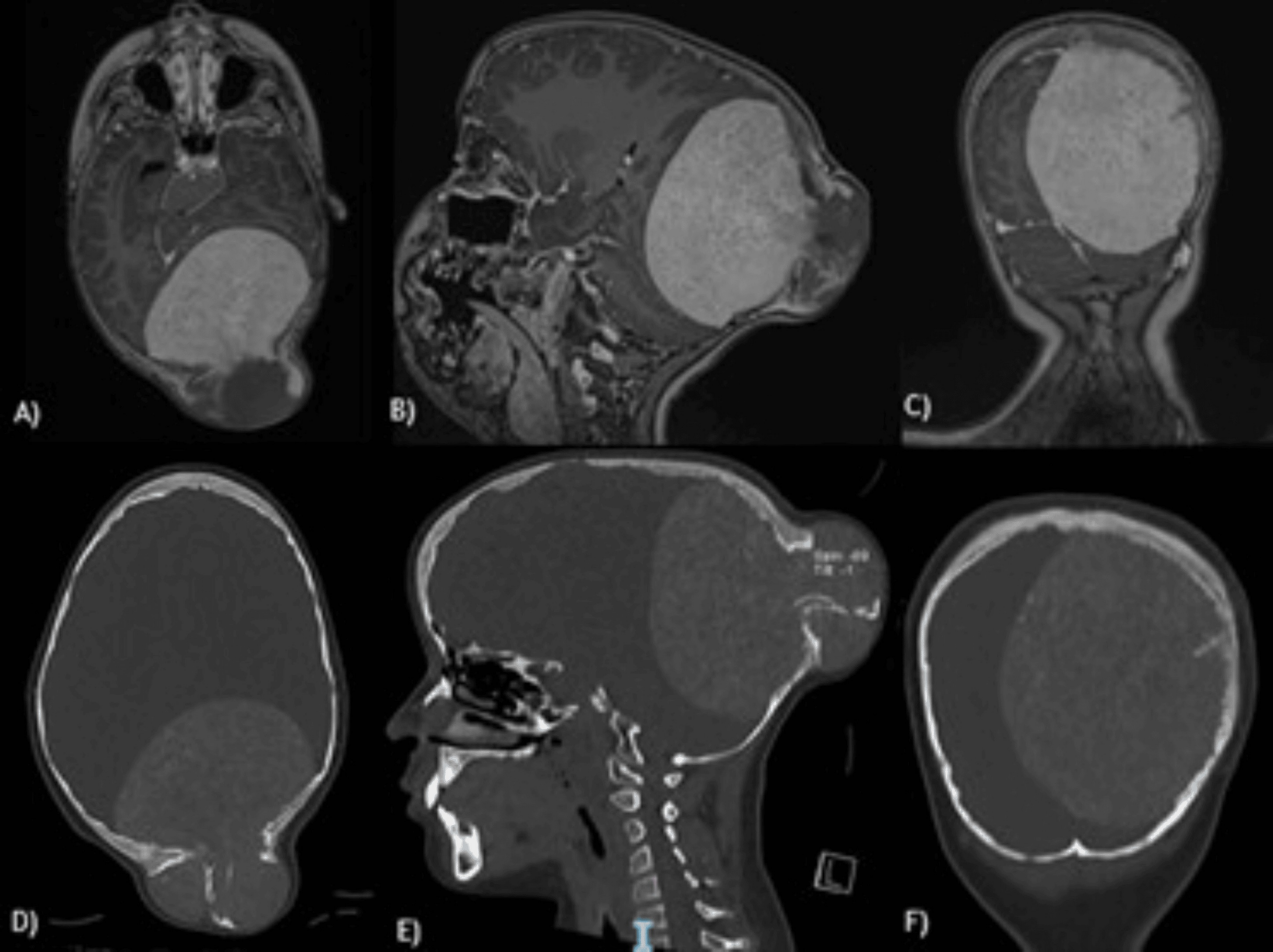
Axial, sagittal, and coronal T1 contrast sequences of the brain MRI and CT images. A) Axial, B) sagittal, and C) coronal T1 contrast sequences of the brain MRI show an extra-axial, heterogeneous, bilobed solid mass that largely enhances with gadolinium contrast in the occipital region of the skull. The mass compresses the brain tissue, especially the occipital lobe and cerebellar parenchyma, in the axial, sagittal, and coronal planes, respectively. Additionally, the sagittal superior sinus, torcula, straight sinus, and transverse sinus - especially the left one - are in contact with the tumor. D) Axial, E) sagittal, and F) coronal brain CT bone sequences show the tumor associated with destruction, hyperostosis, and reactive changes in the occipital bone. MRI, magnetic resonance imaging; CT, computed tomography
